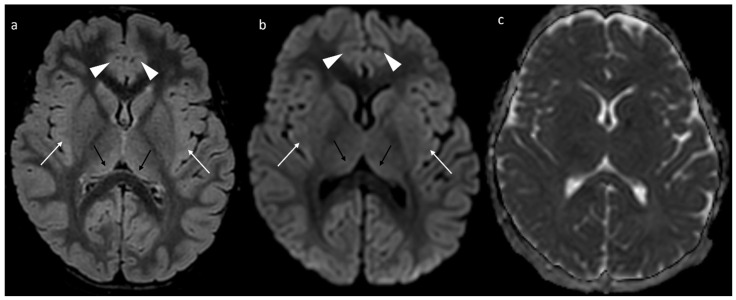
MRI study during status epilepticus shows FLAIR hyperintensity with mild swelling of the cerebral cortex (a), best seen in the insular and cingulate regions (white arrows and arrowheads), and the pulvinar regions of both thalami (black arrows). These regions demonstrate subtle diffusion abnormalities, with increased DWI signal (b) and without a significant drop in ADC values (c).
